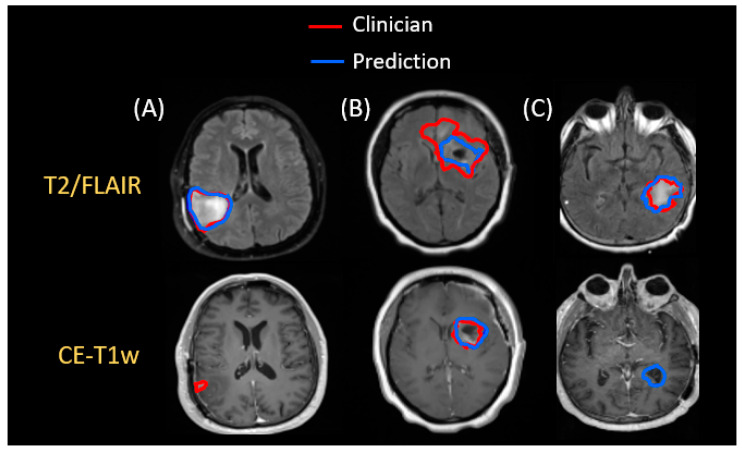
The three worst-performing cases of our 3D Unet model. In (A), the small CE-T1w lesion is missed, possibly being confused for blood vessel. In (B), the model has difficulty capturing the entire extent of hyperintensity from the 3D T2w/FLAIR acquisition, and in (C), the model confuses the ventricle for the shape of a cavity, especially when there are T2w/FLAIR edema in the same region.
